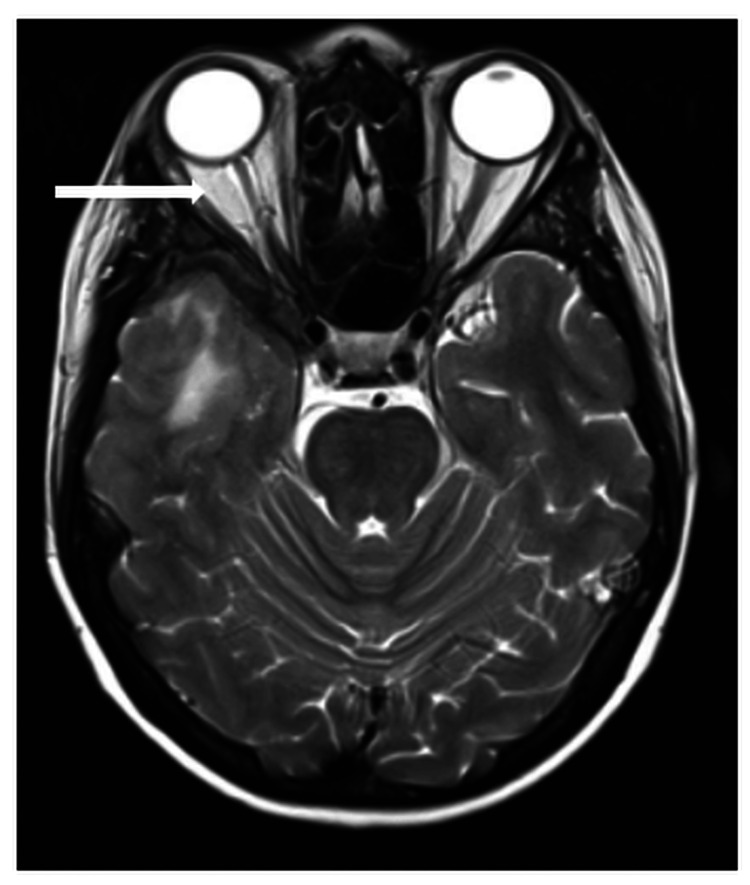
An MRI of the brain (axial view, T2 sequence) showed the presence of enhancement involving the right optic nerve.
